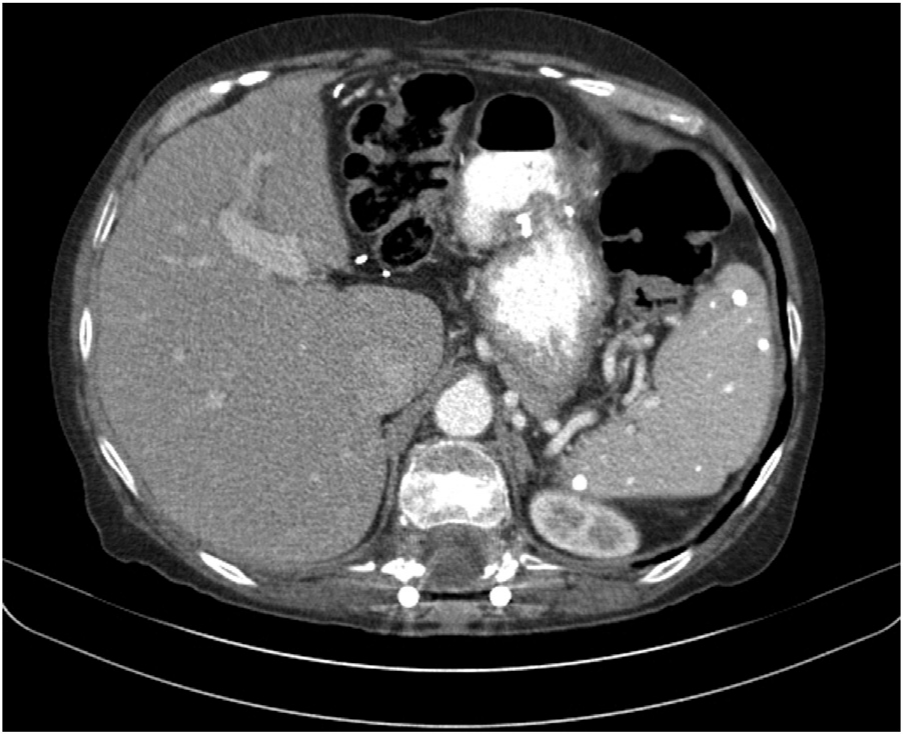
Abdominal CT scan showing a thickened elongated jejunal stricture past the gastrojejunostomy.
